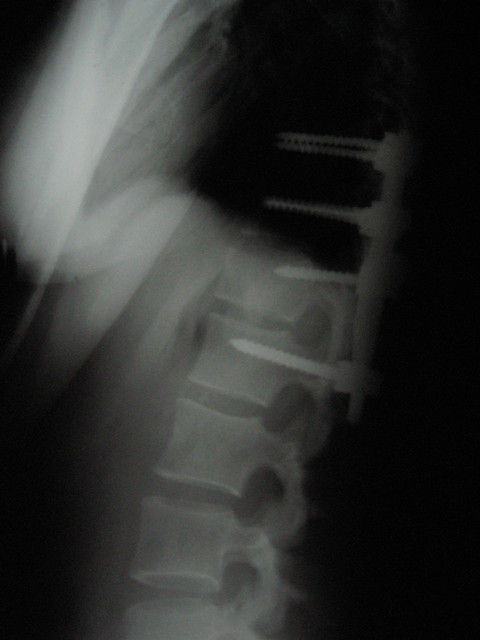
Lateral x-rays post-stabilization.
